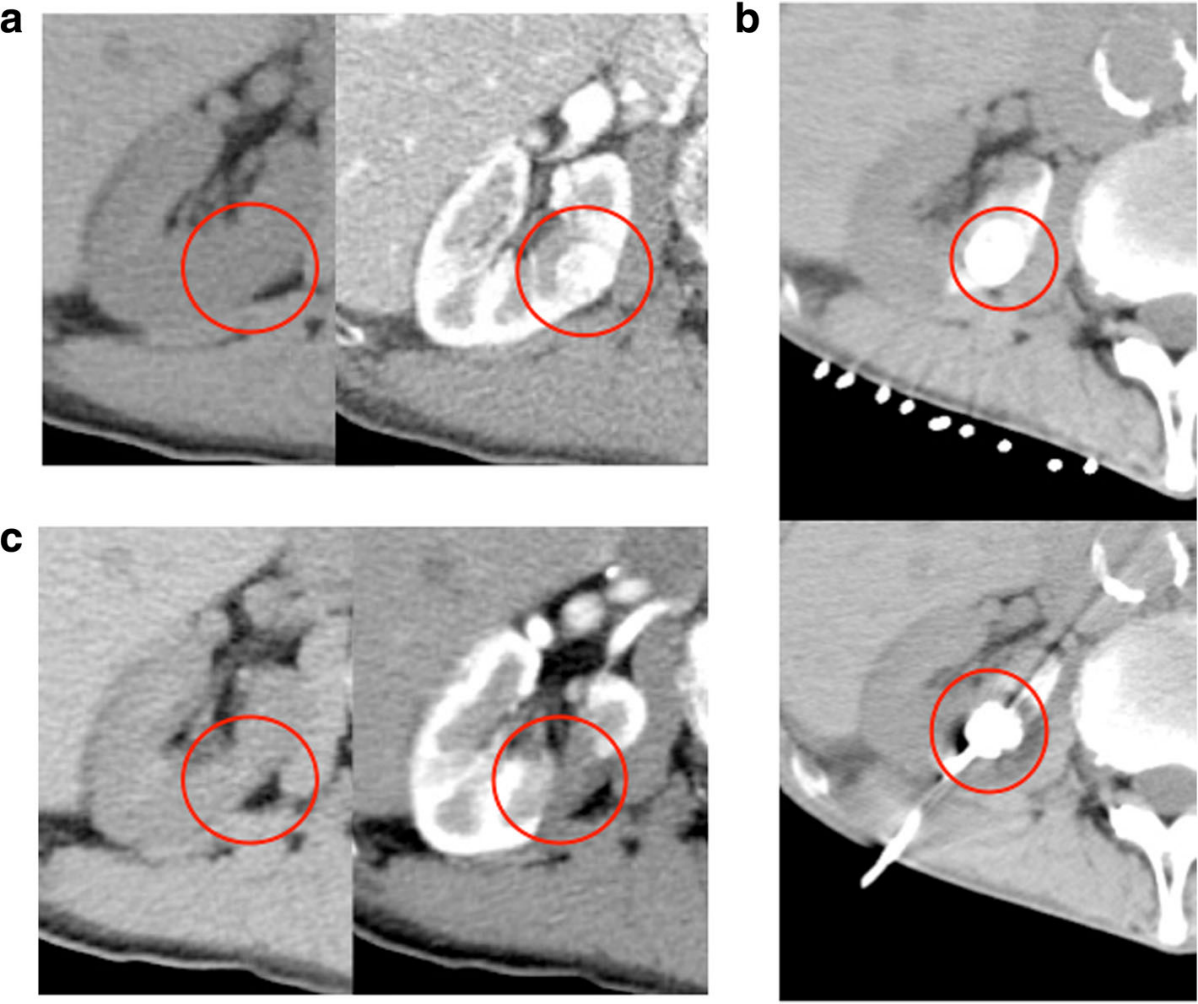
A case of cryoablation with preoperative lipiodol marking. The red circle indicates the primary tumor. a The right renal tumor was detected by a preoperative dynamic computed tomography (CT) scan. b The tumor was easily detected by intraoperative plain CT after lipiodol marking. c A postoperative CT scan showed no enhancement in the cryoablated area. A complete response was achieved by cryoablation according to the mRECIST criteria
